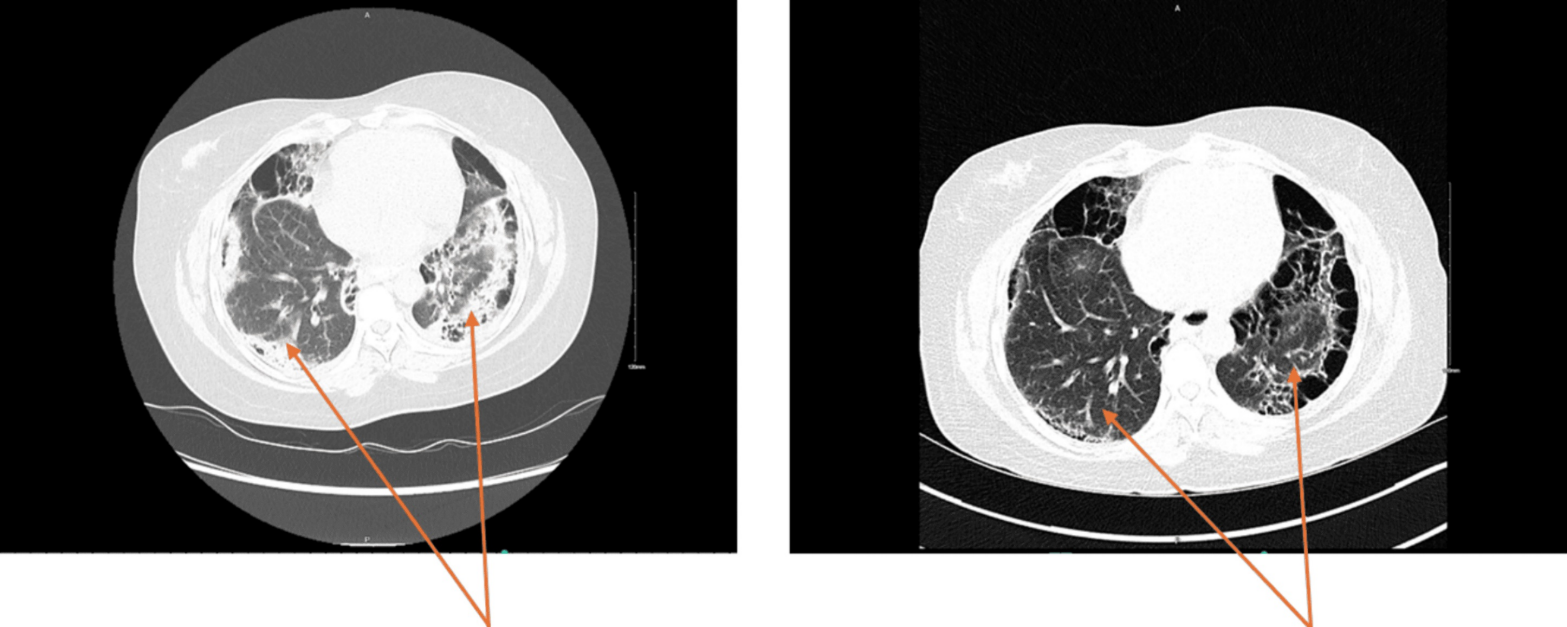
Comparison of High-Resolution Chest CT Scans in a Patient With Systemic Sclerosis-Related Interstitial Lung Disease (SSc-ILD) Before and After Treatment Left: Baseline chest CT showing extensive bilateral lower lobe reticulation, honeycombing, and ground-glass opacities, suggestive of active fibrosing interstitial lung disease. Right: Follow-up CT after three months of immunosuppressive and antifibrotic therapy demonstrating interval improvement in ground-glass opacities and stabilization of fibrotic changes, indicating a partial response to treatment.
